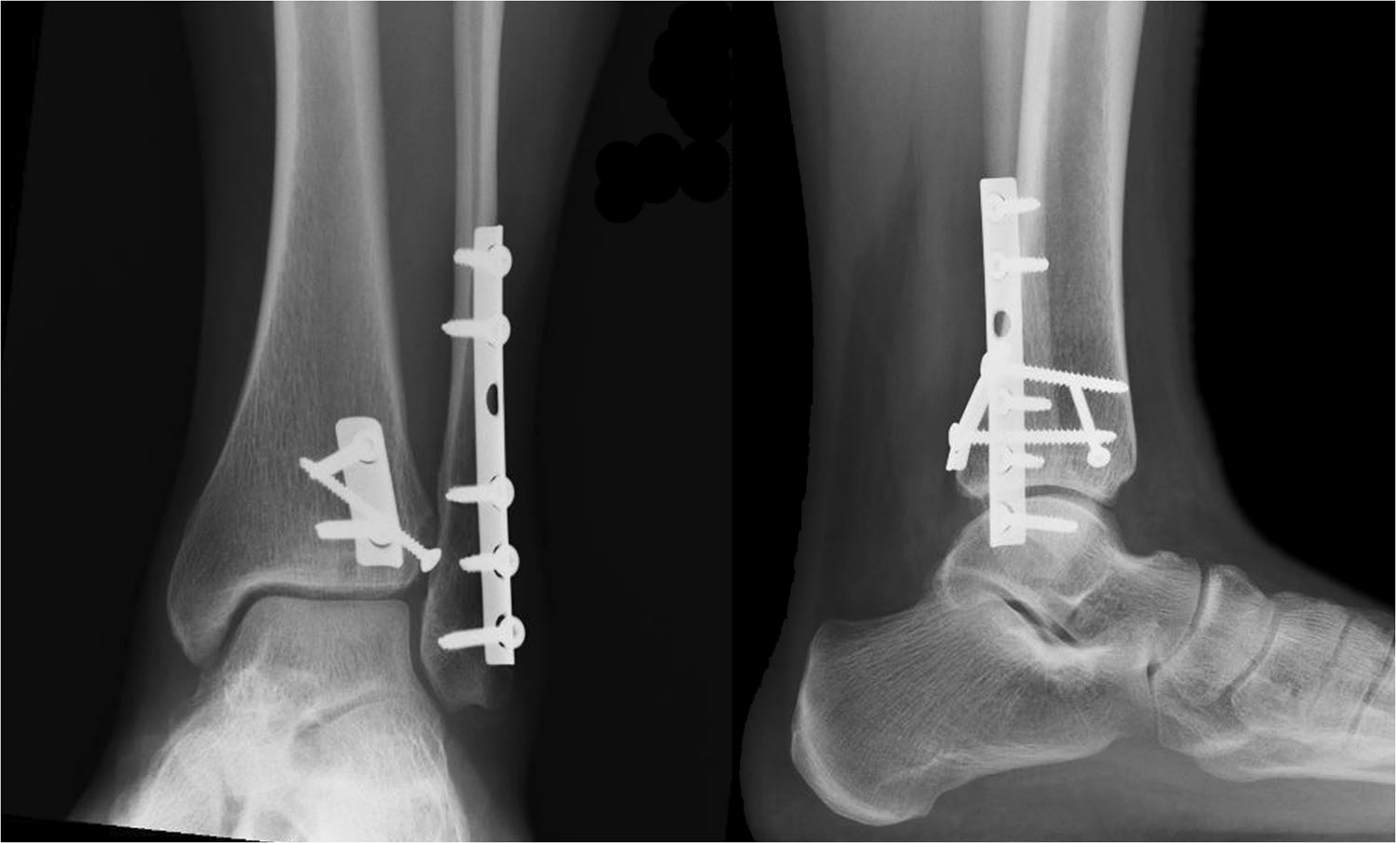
Weight bearing X-rays at 1 year
